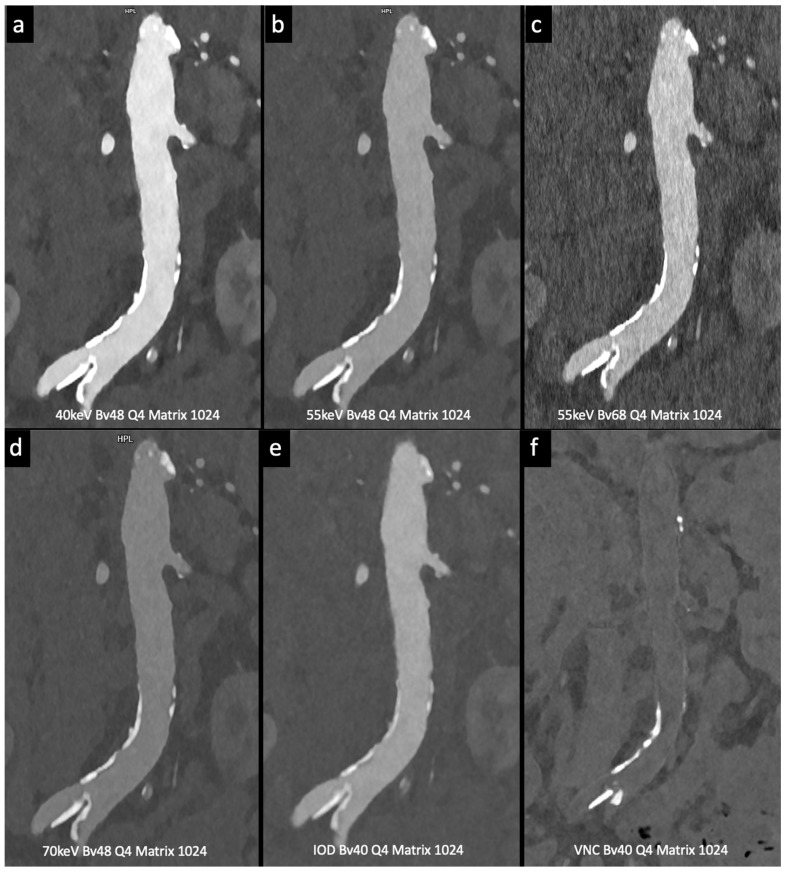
Comparison of image quality with photon-counting CT and standard contrast media. Abdominal aortic lumen with wall calcification coronal images. High Resolution images (Matrix 1024 × 1024) with different reconstruction kernels (window level W2000, C700): 40 keV Kernel Bv48, Q4 (a); 55 keV Kernel Bv48, Q4 (b); 55 keV Kernel Bv68, Q4 (c); 70 keV Kernel Bv48, Q4 (d); Iodine map reconstruction, kernel Bv40, Q4 (e); Virtual Non Contrast VCN reconstruction (f). Department of Radiology, Fondazione Toscana Gabriele Monasterio, Pisa, Italy.
